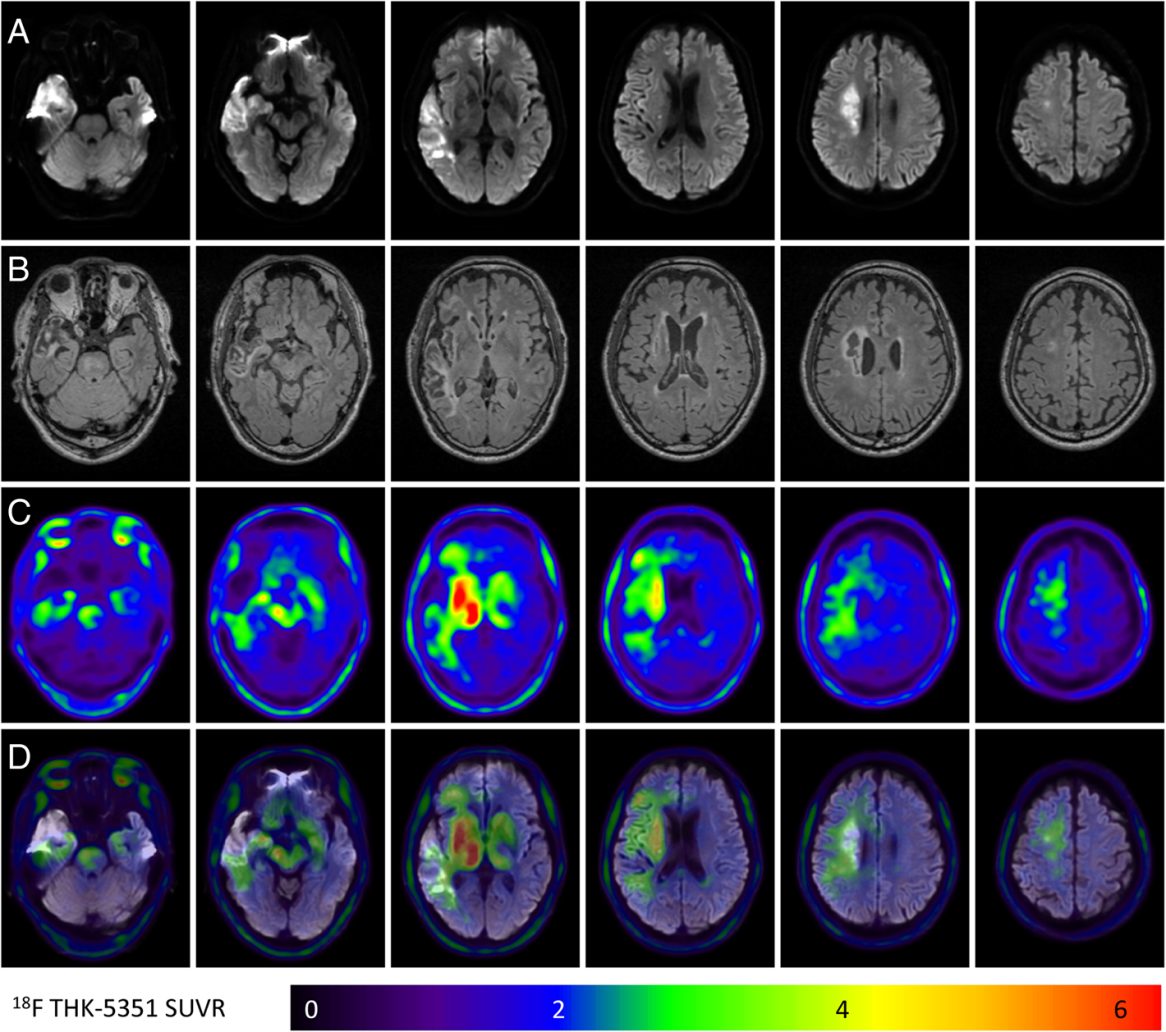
^18^F-THK-5351 retention patterns in an ischemic stroke patient (case 6). a The acute ischemic location on diffusion-weighted imaging (DWI). b, c The FLAIR images and ^18^F-THK-5351 PET images taken 3 months after stroke, respectively. d The fused DWI and ^18^F-THK-5351 PET images. Common off-target binding of the ^18^F-THK-5351 is observed in the bilateral striatum, thalamus, and brainstem. Additionally, the ^18^F-THK-5351 retention is asymmetrically increased in the remote and peri-infarct areas and decreased in the infarct core
